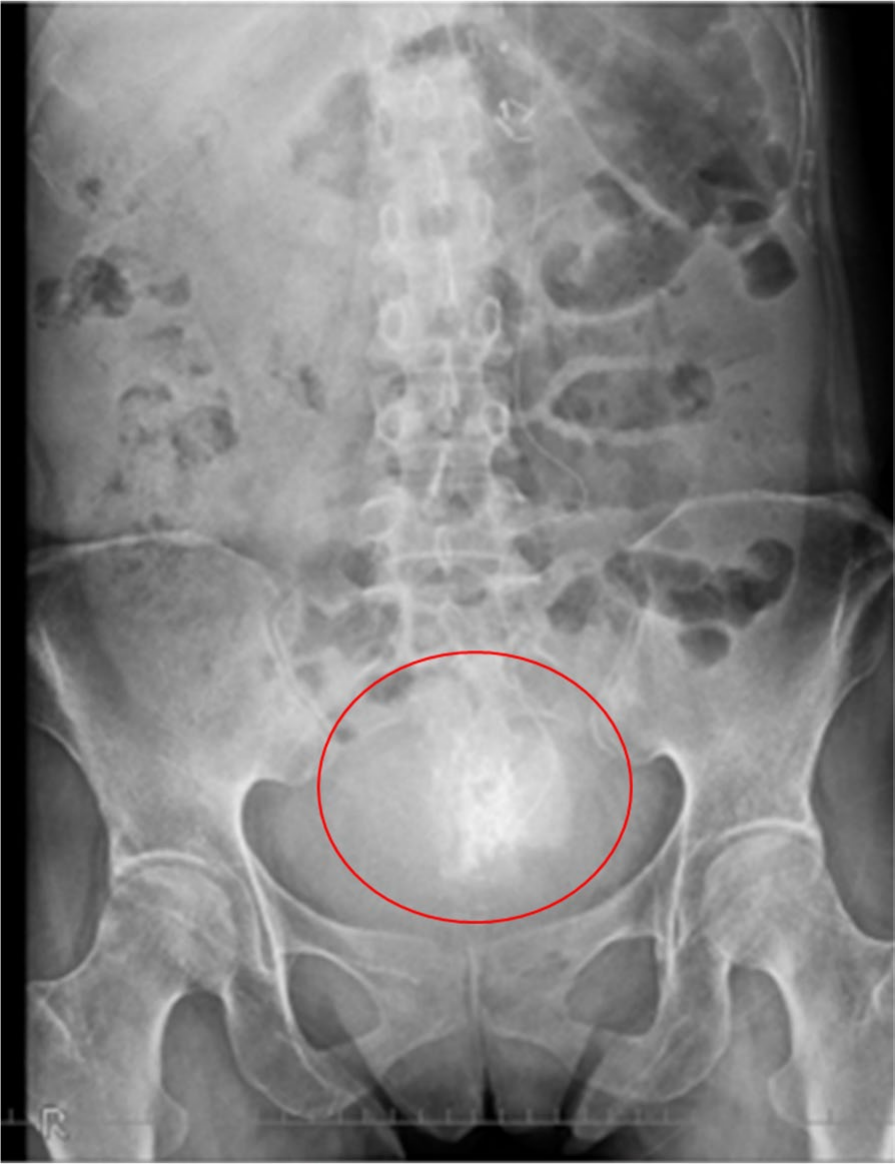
X-ray image injected with contrast agents the next morning after the sacral epidural catheter was placed. Contrast findings are seen around the S4/5 level
